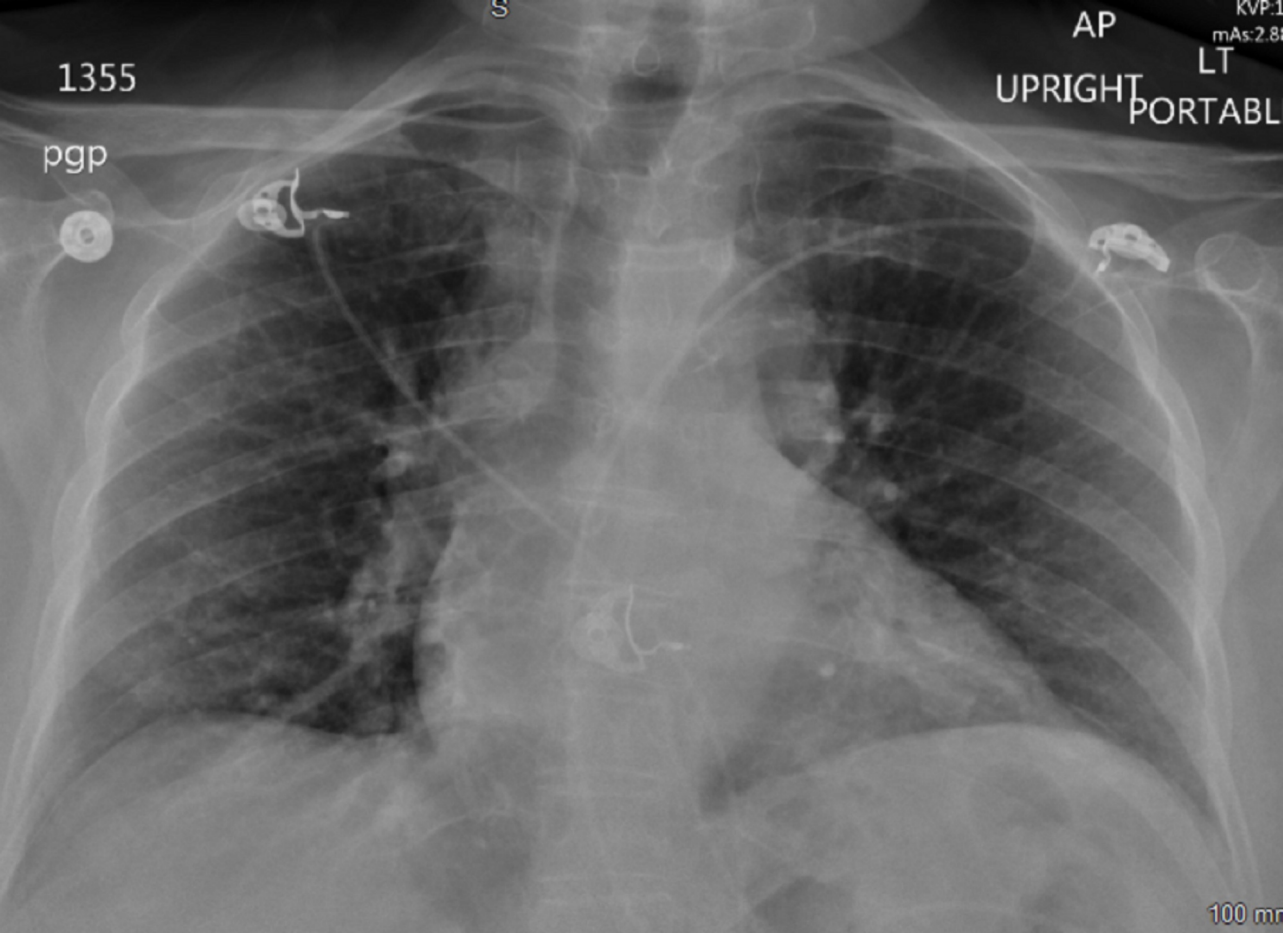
Chest X-ray showing no significant abnormalities and reported as normal
